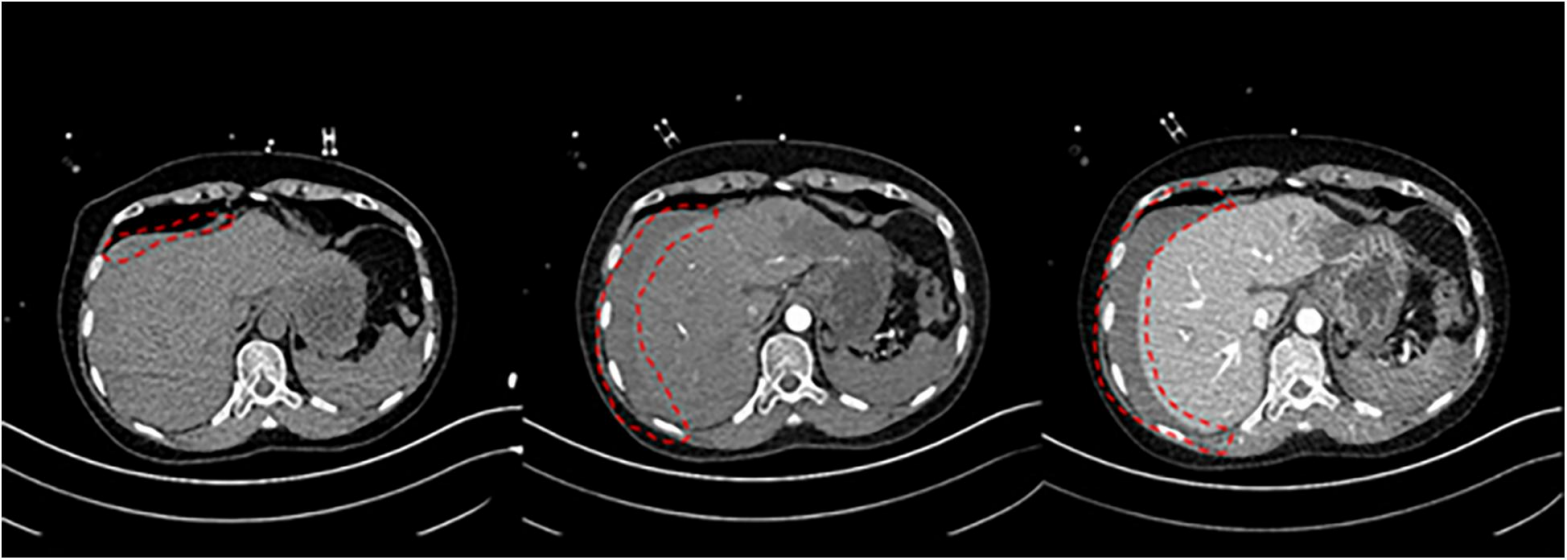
Non-contrast CT of the abdomen, arterial phase, venous phase.
